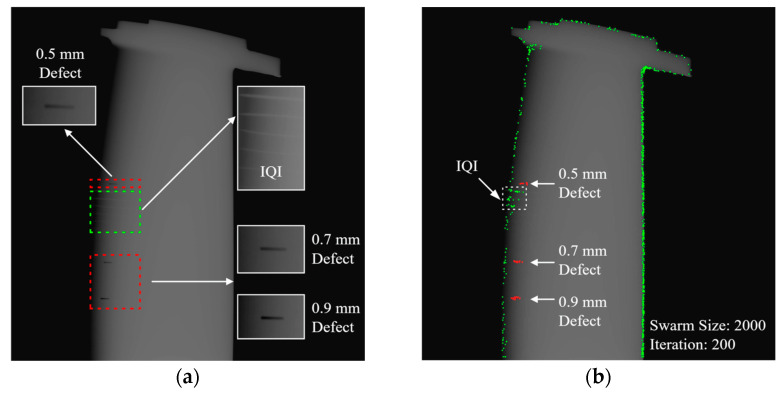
Radiographic image of test blade in (a) raw image; (b) FS-PSO process result.
